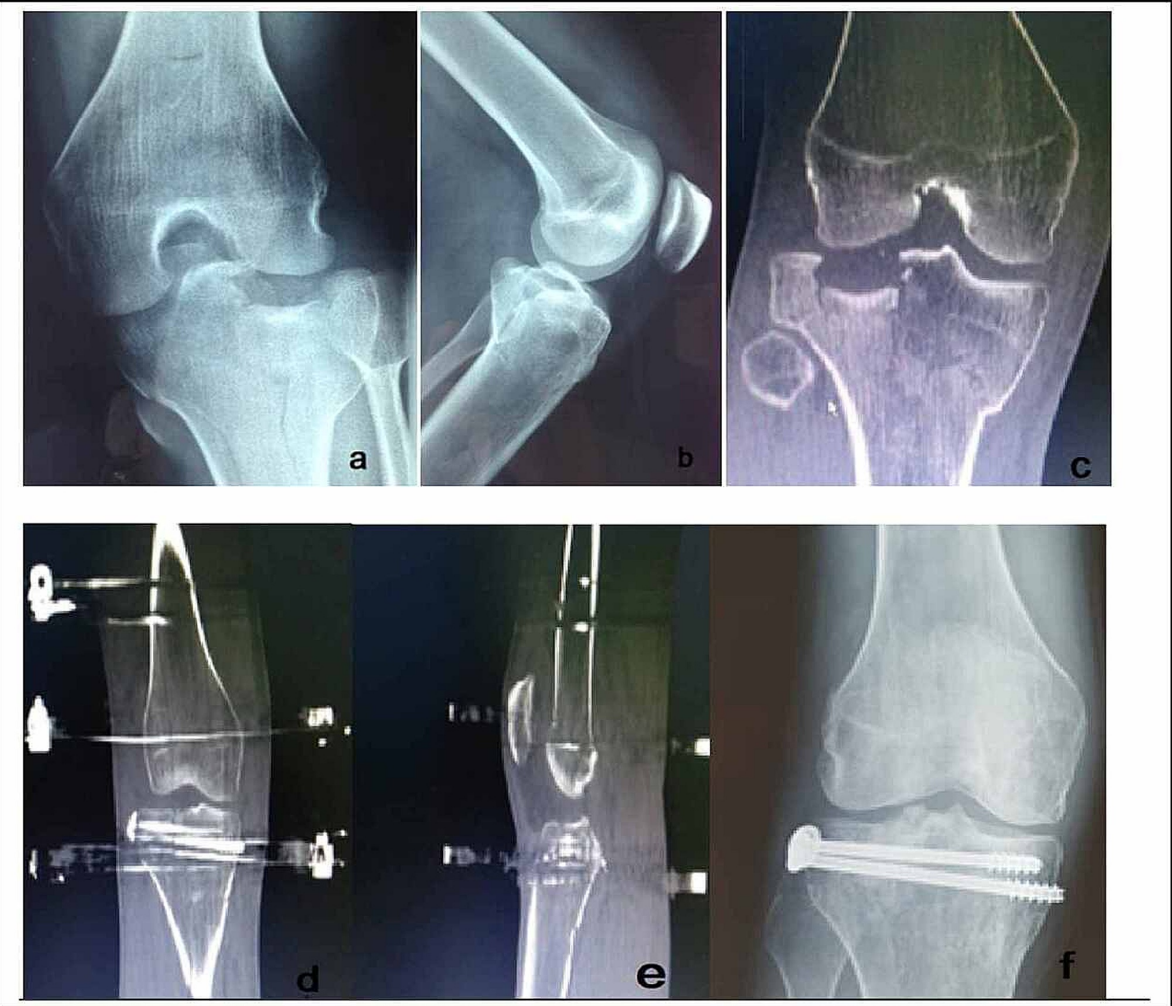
An 18-year-old man with a displaced bicondylar tibial plateau fracture. (a, b) Anteroposterior and lateral preoperative X-ray (c) Preoperative axial CT scan of the knee joint. (d) Postoperative CT scan (axial view) following mini-open reduction of the articular surface and application of an Ilizarov frame with knee bridging. (e) Postoperative sagittal CT scan view of the same patient. (f) Anteroposterior X-ray 5 months postoperatively.
